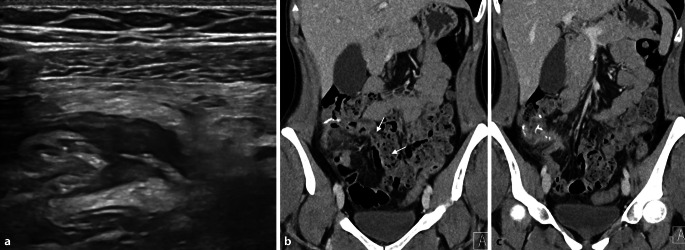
Die Sonographie des rechten Oberbauchs (a) mit einem hoch auflösenden Linearschallkopf zeigt das blinde Ende der Seit-zu-Seit-Enterokolostomie. b,c Venöse Computertomographie des Abdomens mit Nahtmaterial im Bereich der Anastomosenregion, b Verlauf des neoterminalen Ileums (*Pfeile*)
